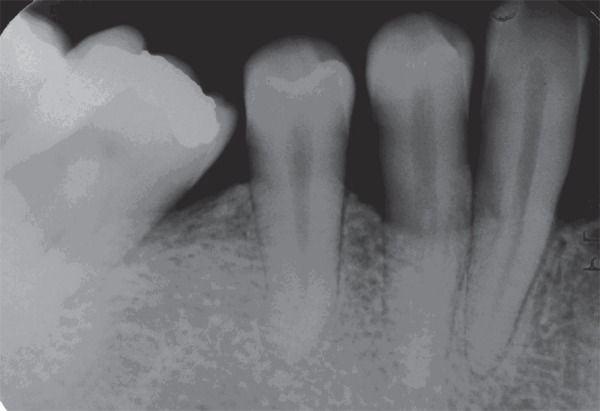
Six-month postoperative radiograph
